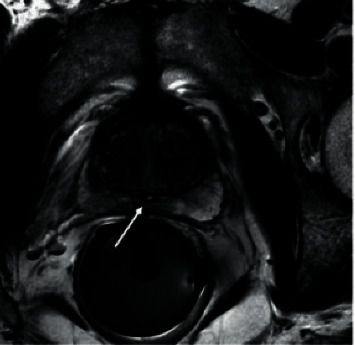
MRI of massive prostatic hyperplasia in the right lobe with hemorrhage at the lower edge of the mass (arrow).
